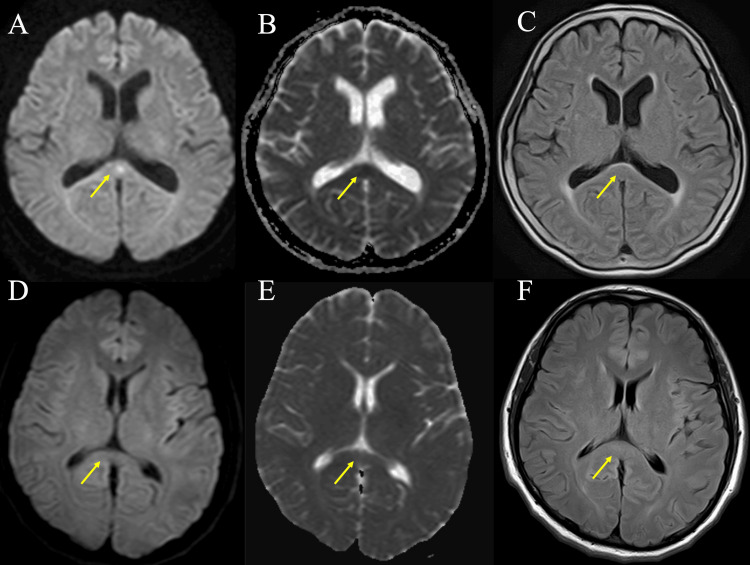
Case 2: MRI findings of CLOCCs (A) DWI shows a high-signal-intensity lesion in the splenium of the corpus callosum (yellow arrow). (B) The ADC map shows a corresponding low-signal-intensity lesion, consistent with cytotoxic edema (yellow arrow). (C) FLAIR imaging shows no significant signal abnormality in the corpus callosum (yellow arrow). (D-F) Follow-up MRI shows complete resolution of the corpus callosum lesion on DWI (D), ADC (E), and FLAIR (F). ADC, apparent diffusion coefficient; CLOCCs, cytotoxic lesions of the corpus callosum; DWI, diffusion-weighted imaging; FLAIR, fluid-attenuated inversion recovery
